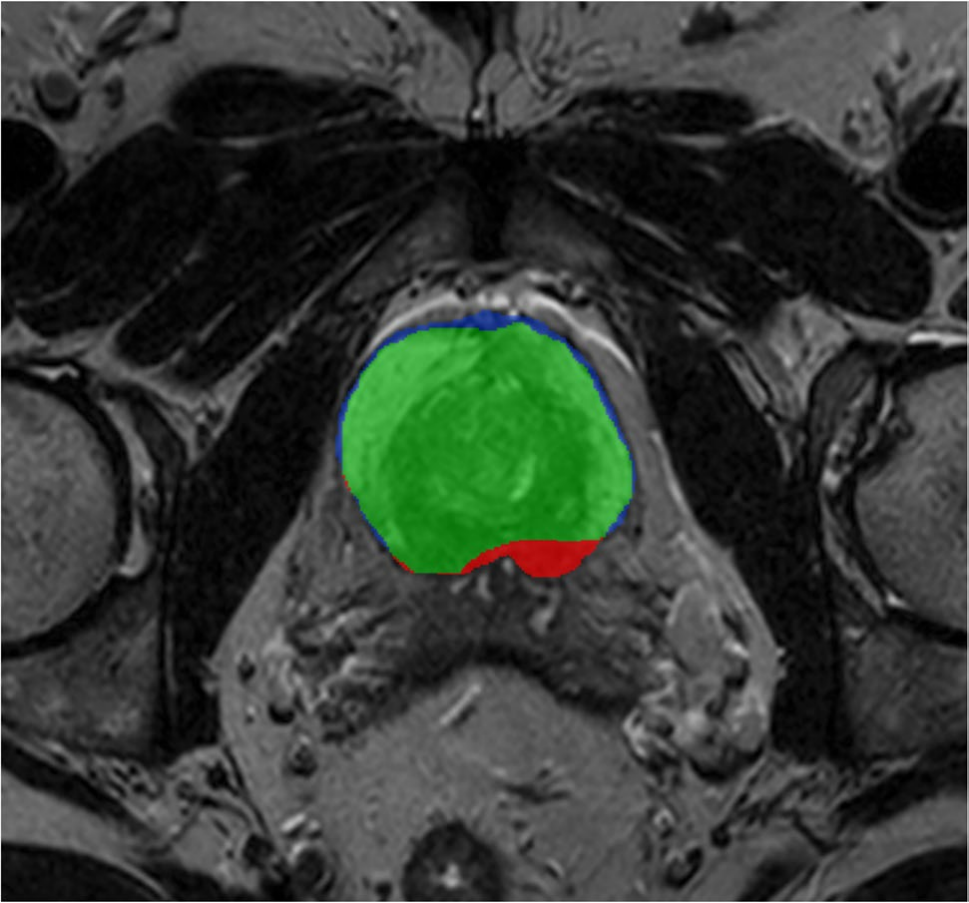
T2-weighted axial image from the feedback session in RECOMIA where green color shows segmented areas in agreement, blue color areas segmented by the study participant only, and red color areas segmented by the expert only
